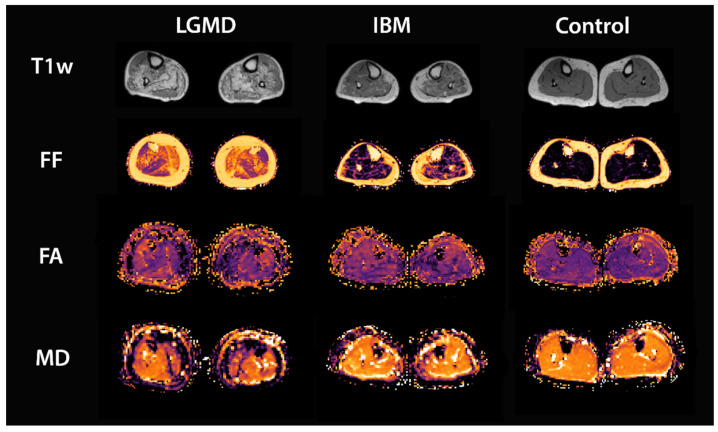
Example images of the applied MRI sequences: T1w, mDixon fat fraction (FF), FA and MD for lower legs of an LGMD, an IBM patient, and a healthy control.
